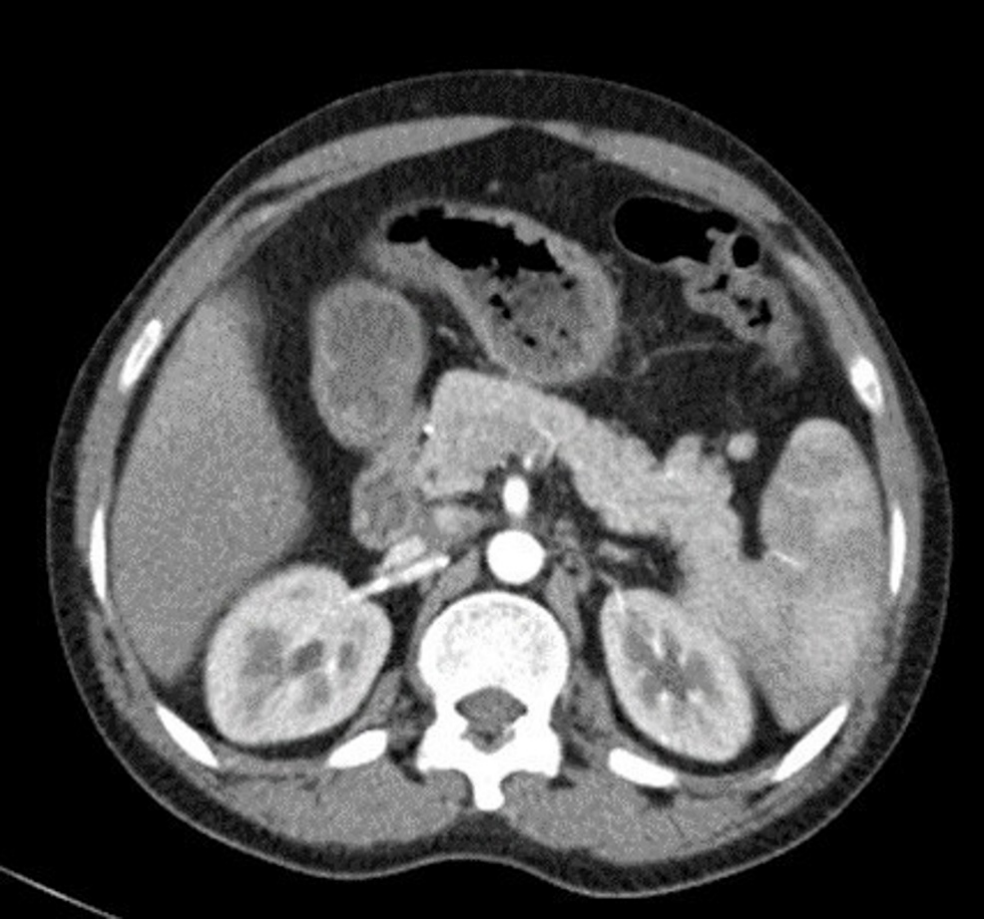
Axial enhanced CT showing resolution of the periureteric fibrotic changes and mild hydronephrosis.
